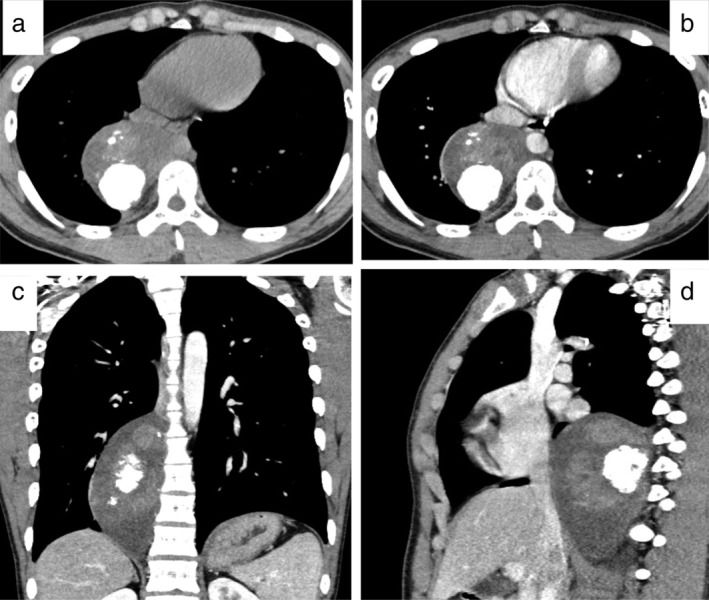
(a) Chest computed tomography showed a right‐sided posterior mediastinal mass with aggregated and nodular calcification. (b) There were enhanced areas within the mass on axial contrast computed tomography. (c and d) Coronal computed tomography showed that the tumor extended along the right vertebral area.
